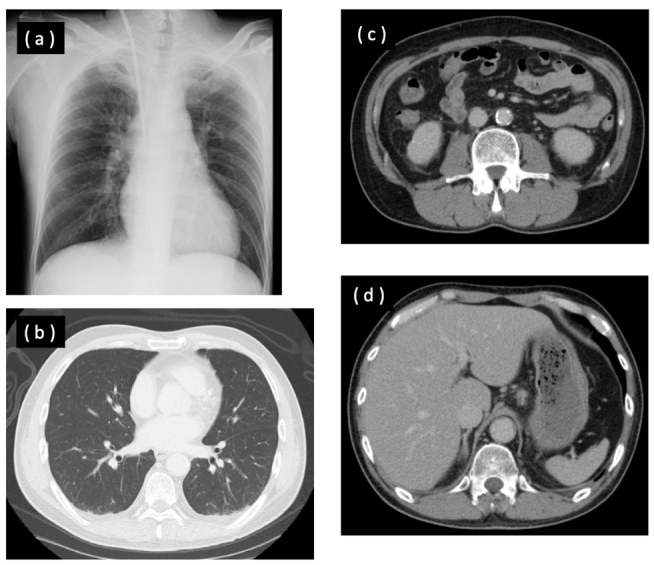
(a) Chest roentgenogram on arrival at our hospital showed no abnormal findings. (b) Chest computed tomography (CT) on arrival showed no abnormal findings. (c) Abdominal CT on arrival showed no abnormal findings. (d) Abdominal CT on arrival showed neither splenomegaly nor atrophy of the spleen.
